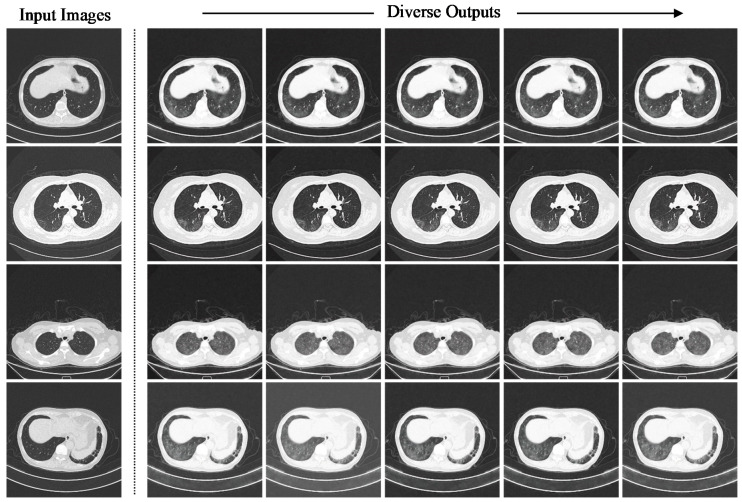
Examples of instance-diverse CAP CT images generated from sRD-GAN.
